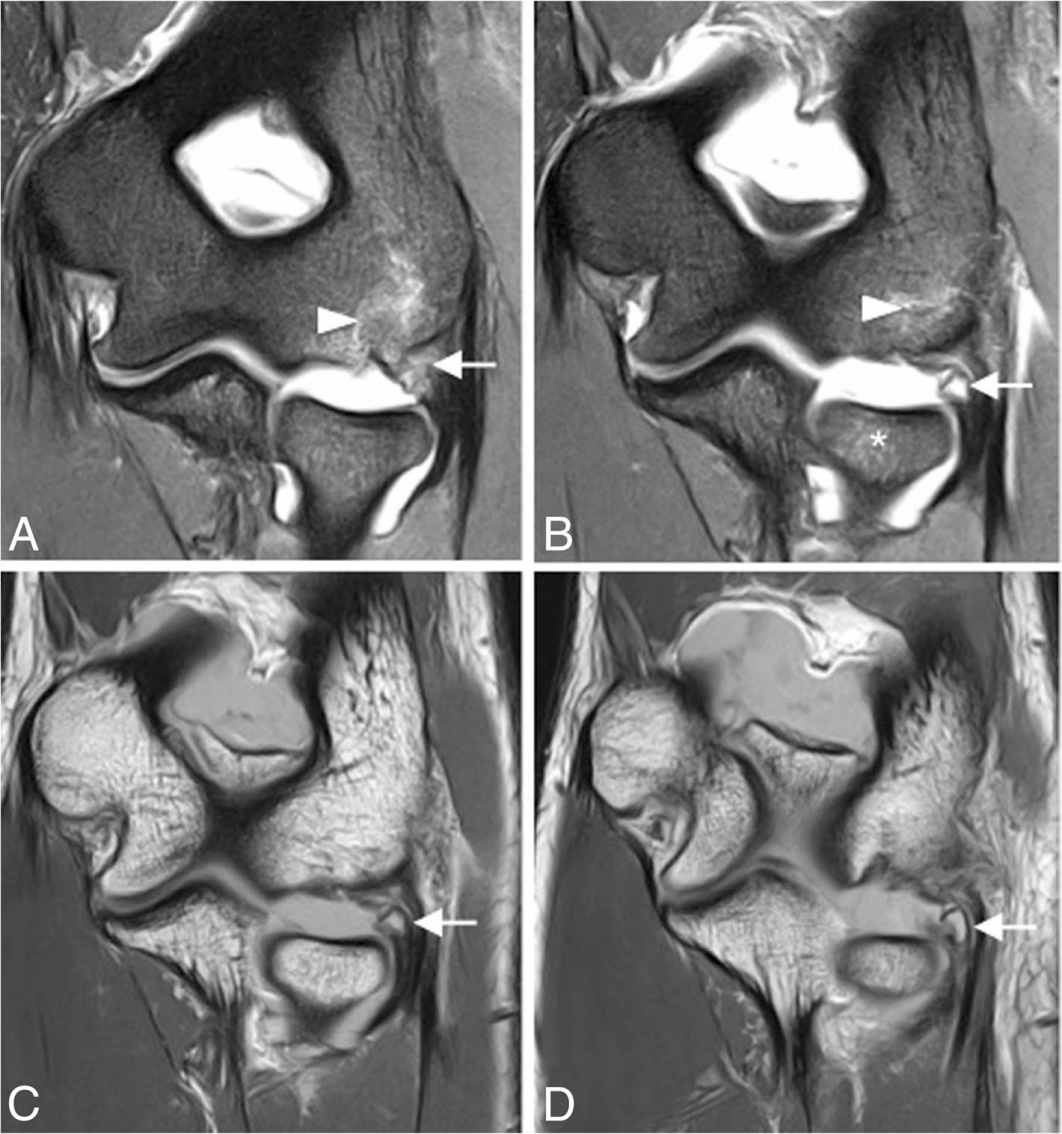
Posterolateral rotatory instability, stage 1. Coronal FS PD-weighted MRI (a, b) and coronal T1-weighted MRI (c, d) showing an avulsion of the proximal lateral ulnar collateral ligament and retraction of the osseous fragment (white arrows). Bone marrow edema is seen in the capitellum (white arrowheads) and radial head (white asterisks)
